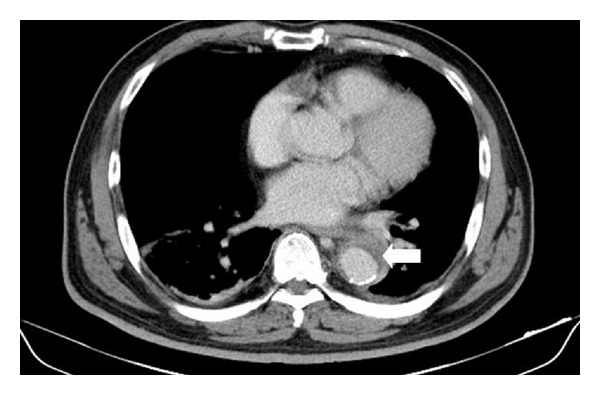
Computed tomography of chest showed aortic intramural hematoma (arrow).
